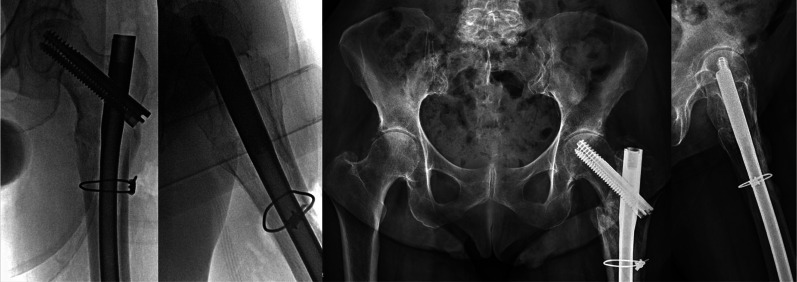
(Left) Anteroposterior (AP) and lateral view of the left hip obtained at the time of revision surgery showing restoration of proximal femoral length and neck shaft angle. (Right) AP pelvis and lateral hip X-ray obtained 6 months postoperatively showing full union of the fracture with no interval shortening
